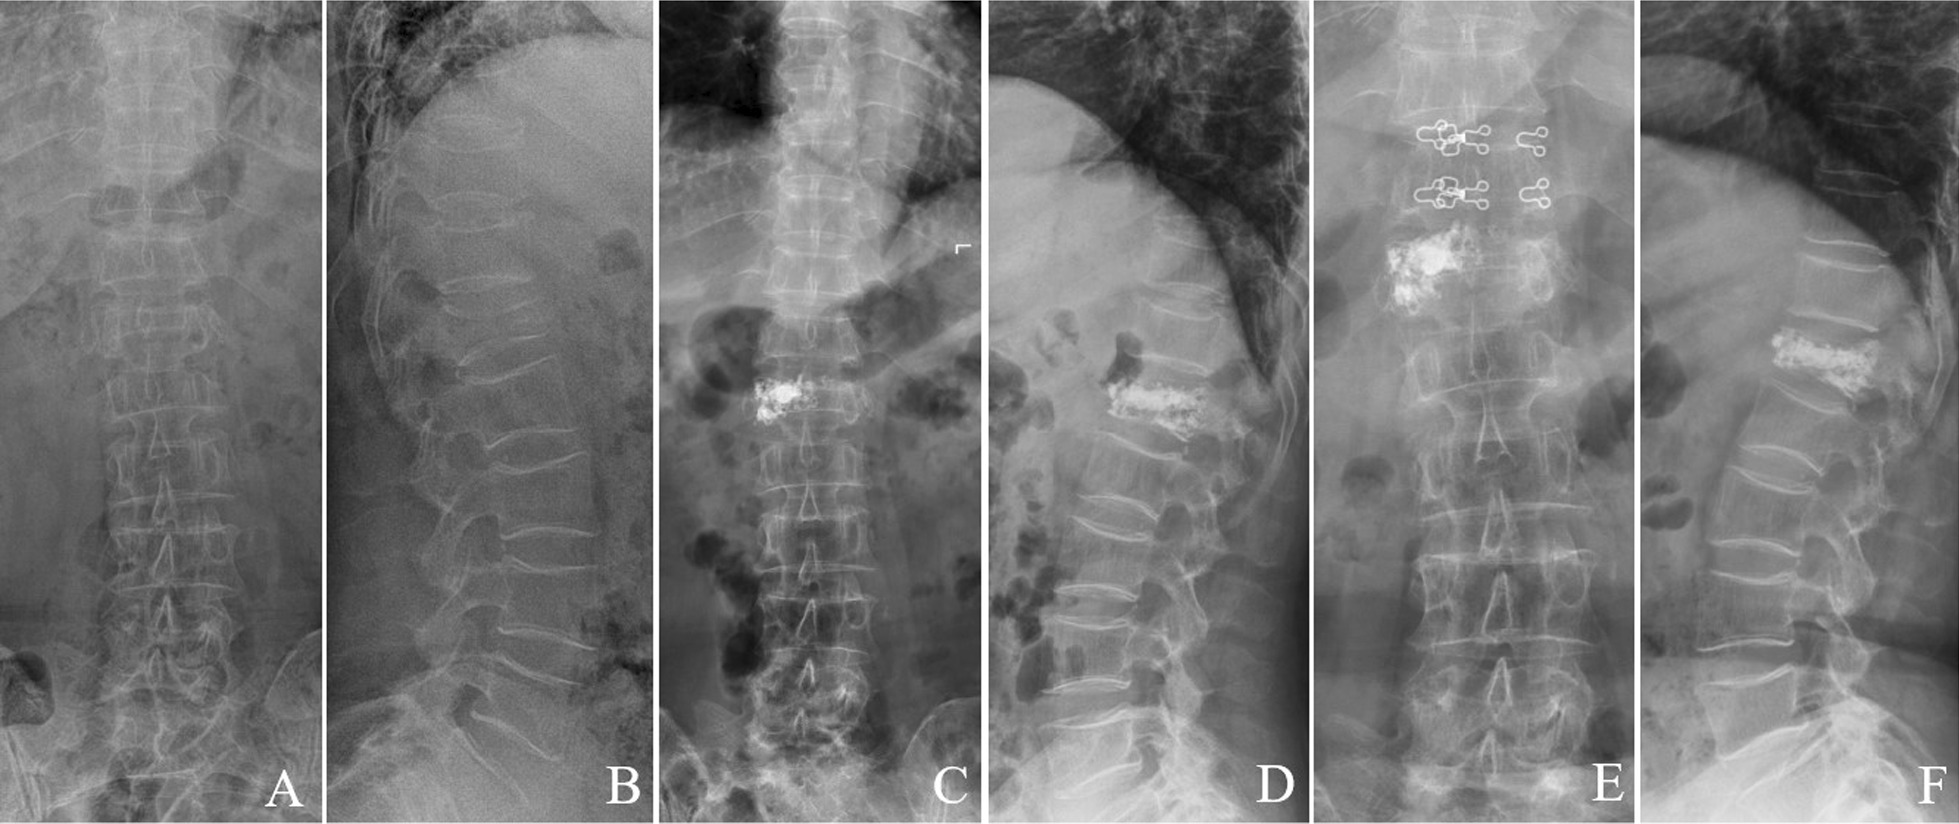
A 70-year-old female with single-level L_1_ OVCF treated by unilateral PKP with traditional bone cement delivery device. A, B, Preoperative anteroposterior and lateral radiographs showed a L_1_ fracture. C, D, one week after operation, the asymmetrical distribution of bone cement. E, F, 12 months after operation: the bone cement remains asymmetrically distributed. The anterior height of the injured vertebra has been diminished, and the kyphotic angle aggravated
